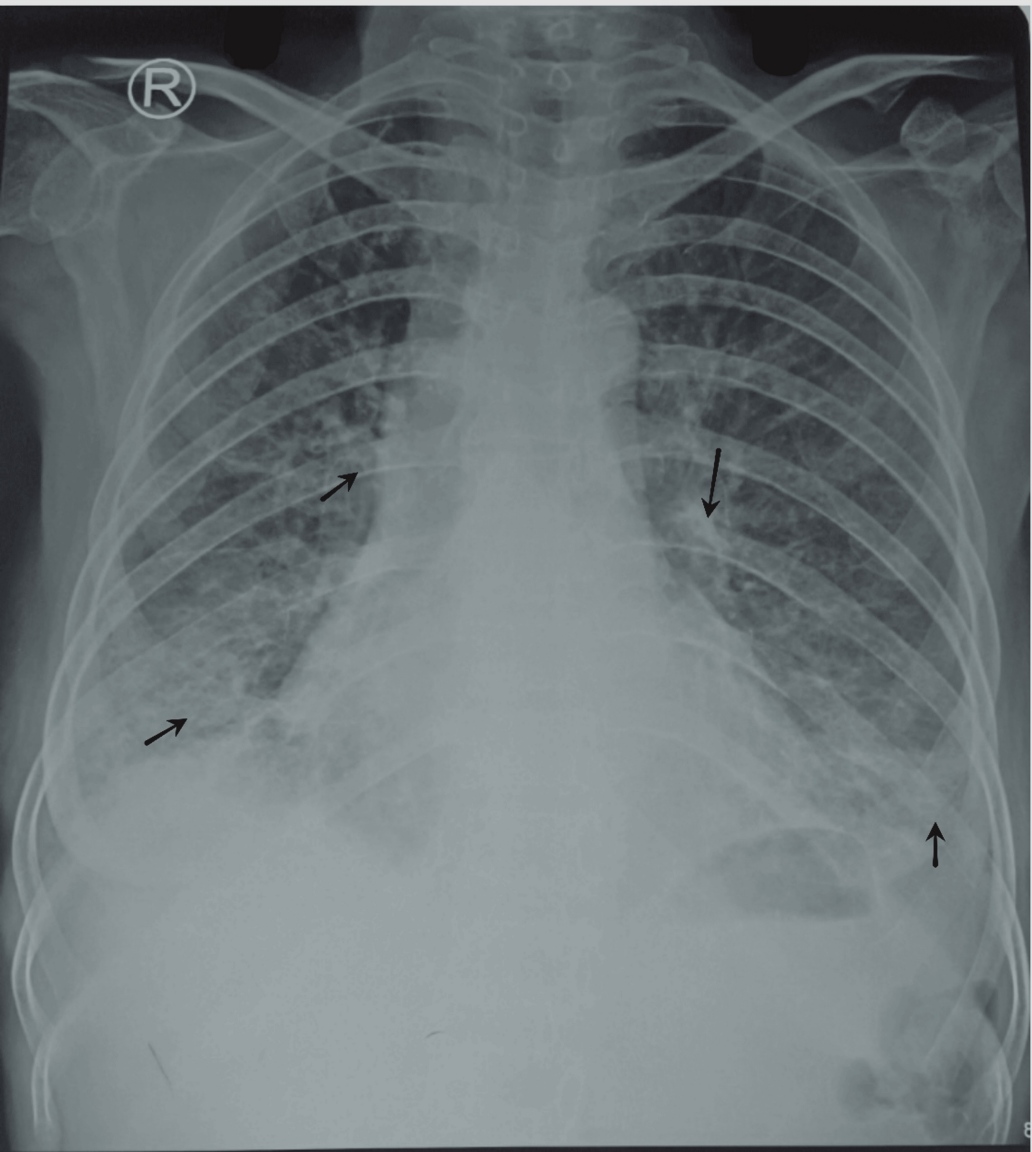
Chest X-ray of the patient showing bilateral hilar fullness and bilateral lower zone haziness.
